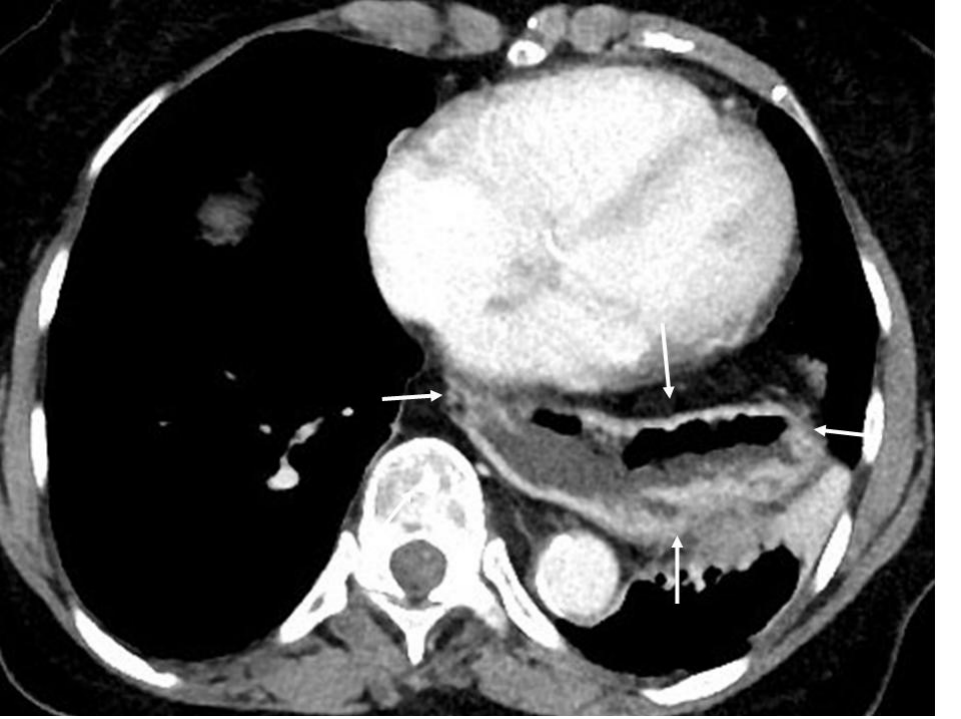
Contrast enhanced CT of the chest of an 84 years old female with complains of chest tightness, regurgitation and dyspepsia for a few years shows a large mixed density mass (arrows) in the left hemi-thorax behind in the heart. The appearance is typical of a hiatus hernia. Density of hiatus hernia is variable but generally contains air and fluid
